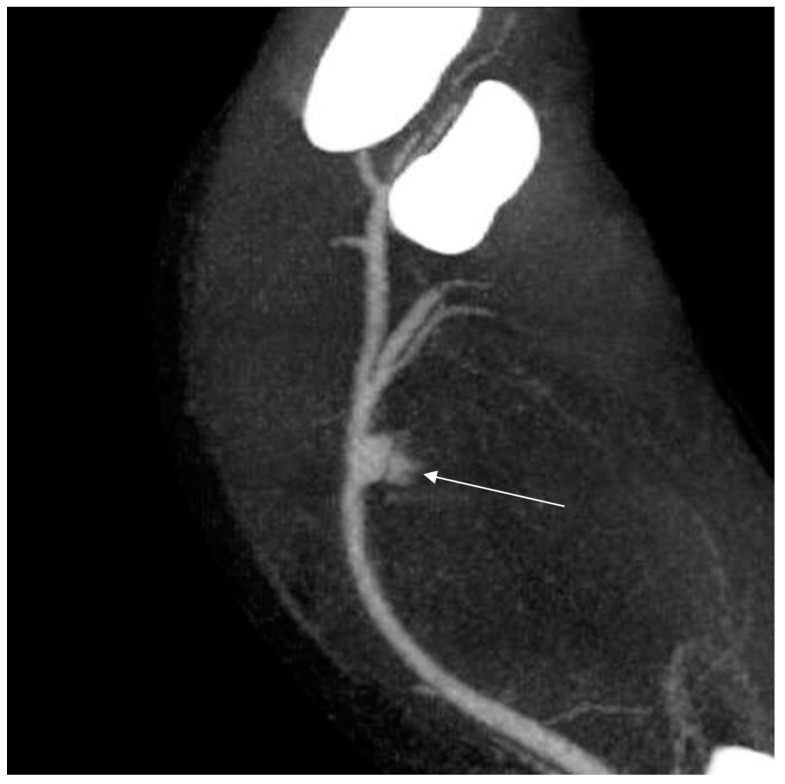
Right upper limb computed tomography angiography. MIP reconstruction. Leakage of the contrast agent beyond the lumen was found, consistent with active bleeding from the injured brachial artery.
